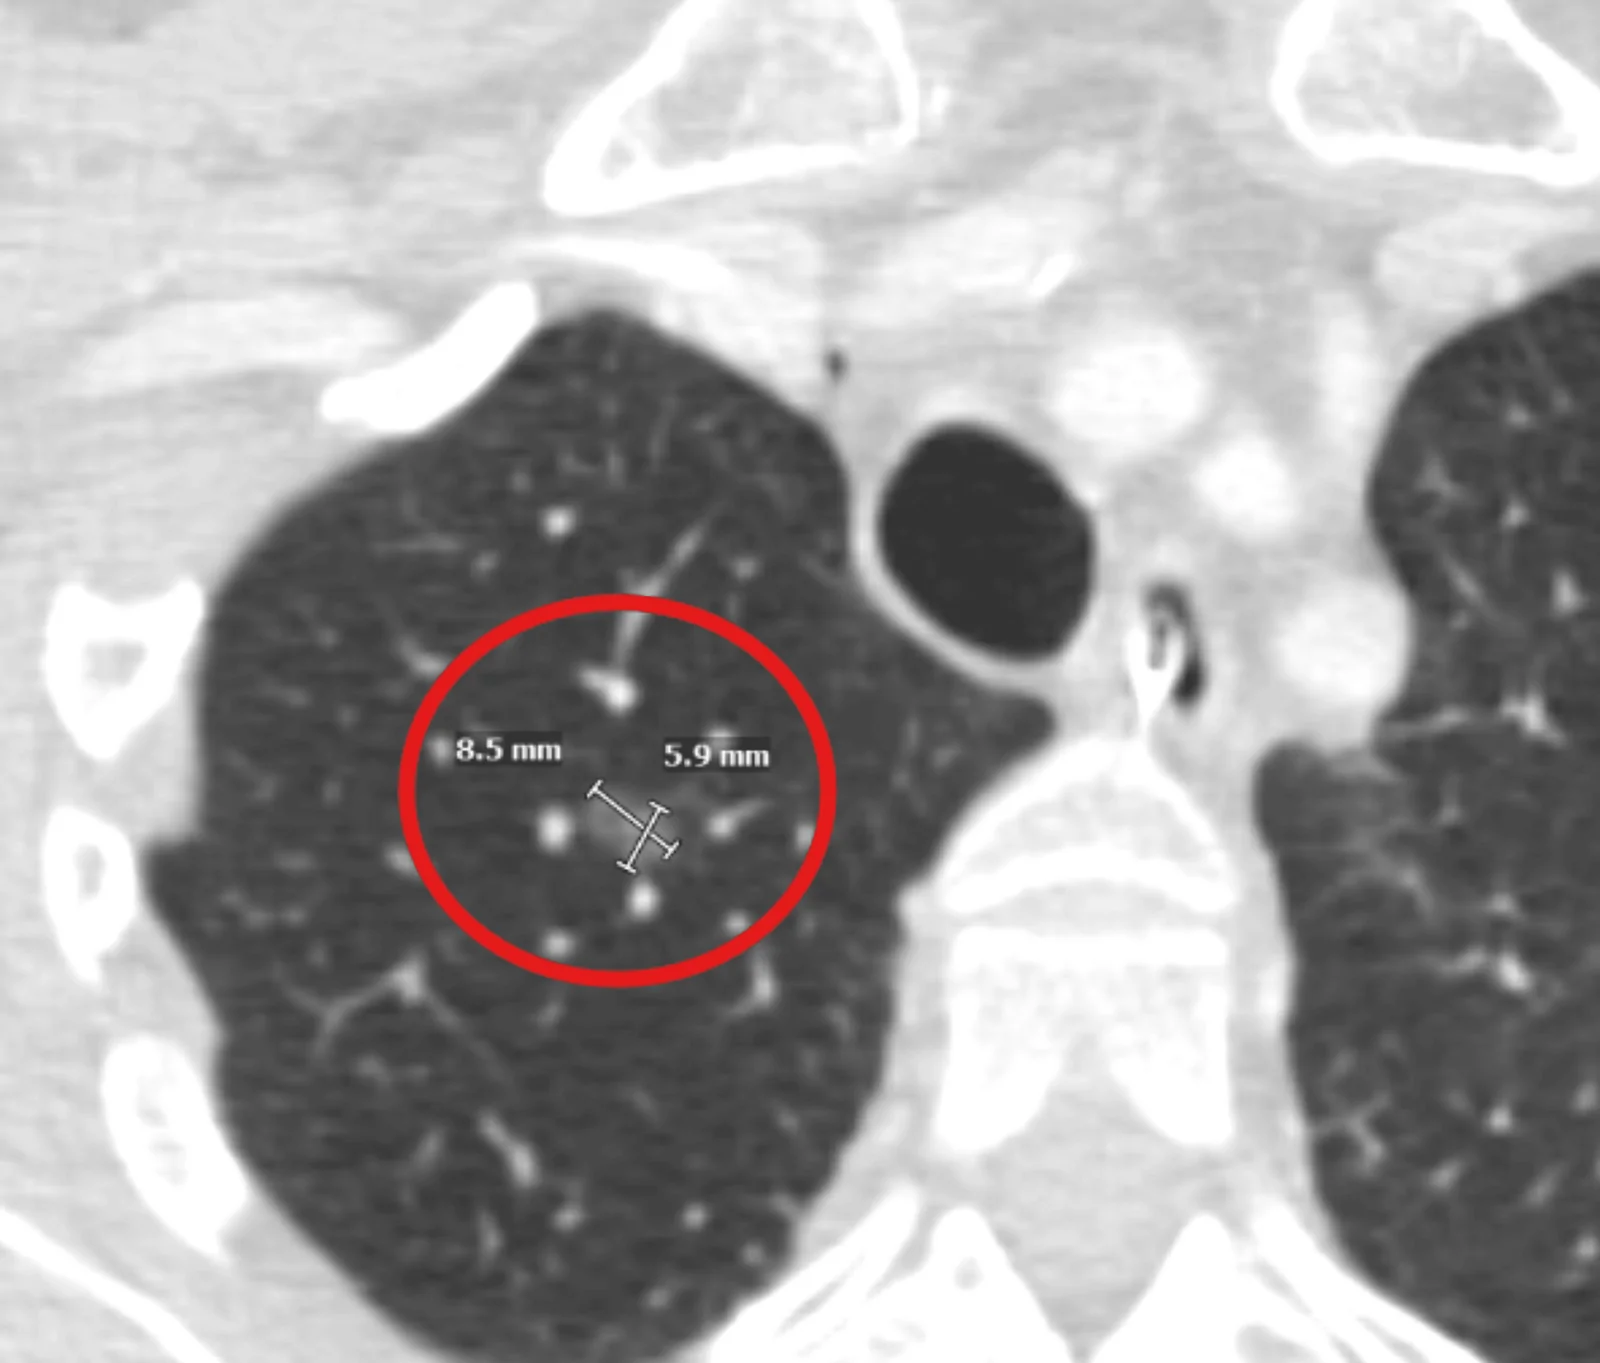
Selected axial contrast-enhanced chest CT image obtained at the time of initial diagnosis, demonstrating a 6 × 9 mm lesion with benign imaging features.
